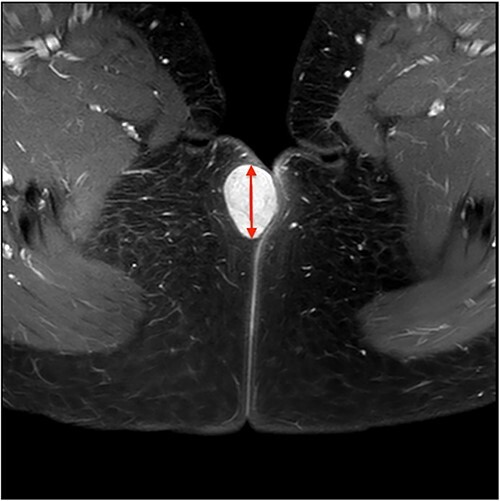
Axial view MRI image of the lesion marked with a red arrow.
